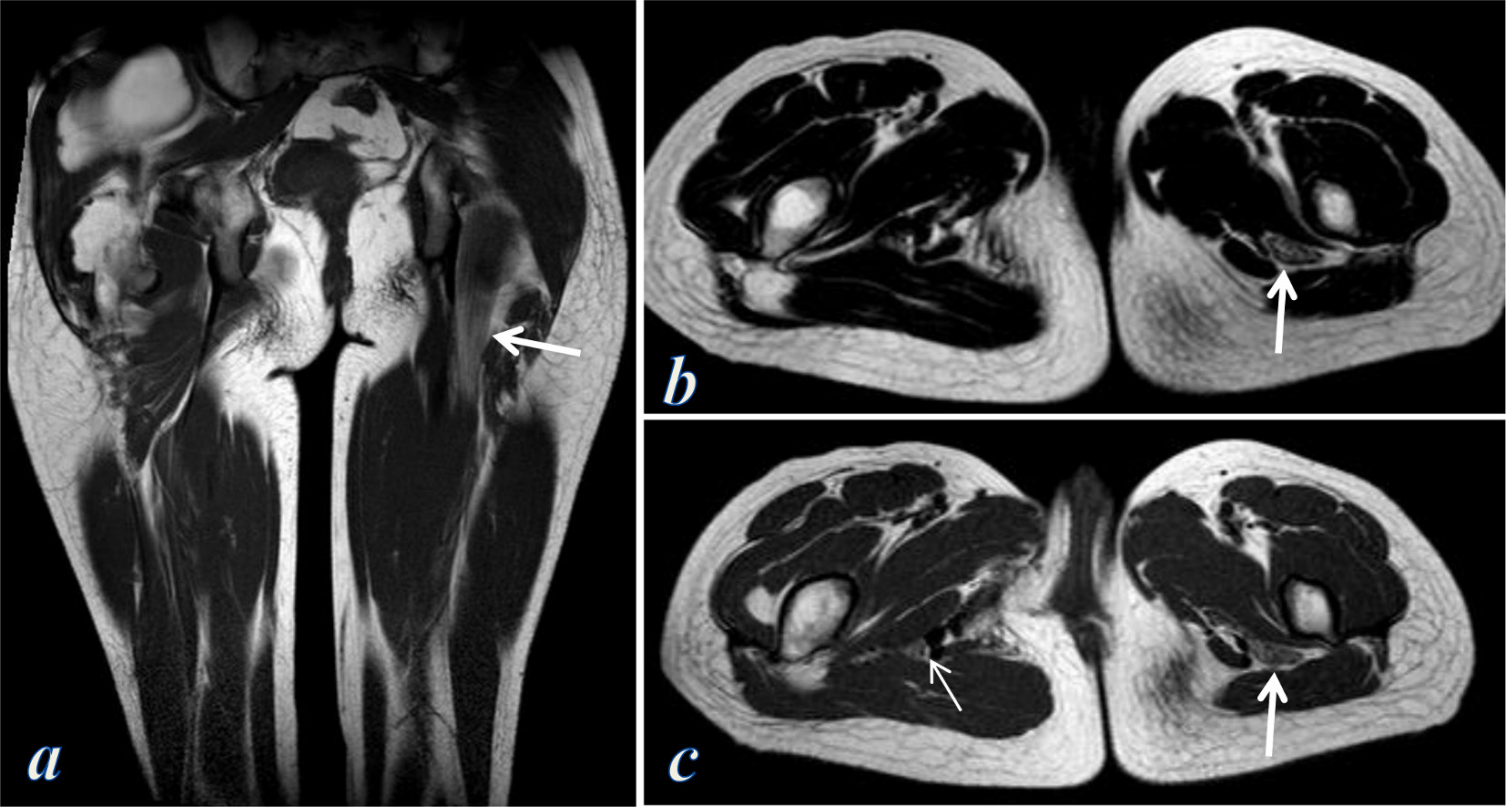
MRI examination of the thighs. (a) Coronal *T*
